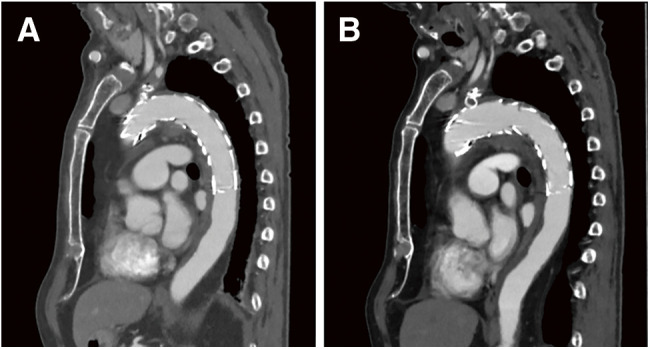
Fig. 3 (A) CT performed in the first postoperative year. (B) CT performed in the second postoperative year. No change in the shape of the descending aorta was observed; however, the curvature of the aortic arch of the stent graft increased slightly, straightening. CT: computed tomography
